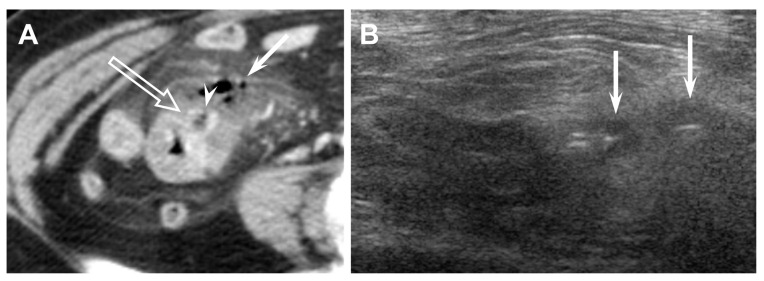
Seventy-year-old female (patient #7) with acute perforated ileal diverticulitis. (A) Axial CT scan shows terminal ileal wall thickening, an outpouching inflamed diverticulum (open arrow) with focal wall defect (arrowhead) at the mesenteric side of terminal ileum, extraluminal air with fluid (arrow), and mesenteric fat infiltration. (B) US scan with linear transducer also reveals extraluminal air bubbles (arrows) representing perforation.
